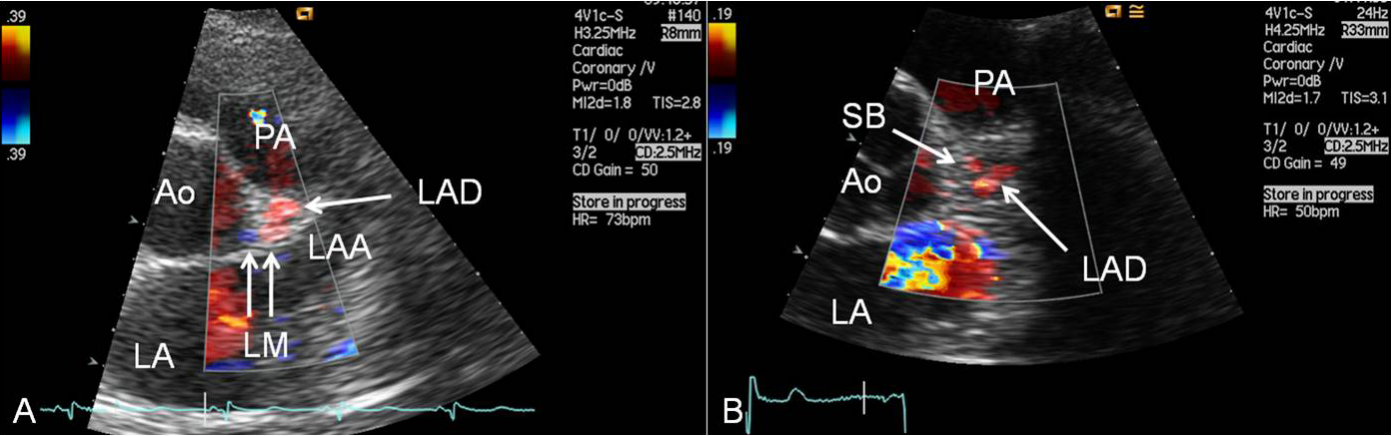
Examples of antegrade coronary artery flow in the LM and proximal parts of LAD. (A) In modified parasternal short-axis view the left main coronary artery (LM) is seen leaving the left sinus of Valsalva and continuing as the left anterior descending coronary artery (LAD) turning slightly towards the transducer. (B) In modified parasternal short-axis view the first septal branch (SB) is seen leaving the LAD. Ao = aortic root; LA = left atrium; LAA = left atrial appendage; PA = pulmonary artery.
